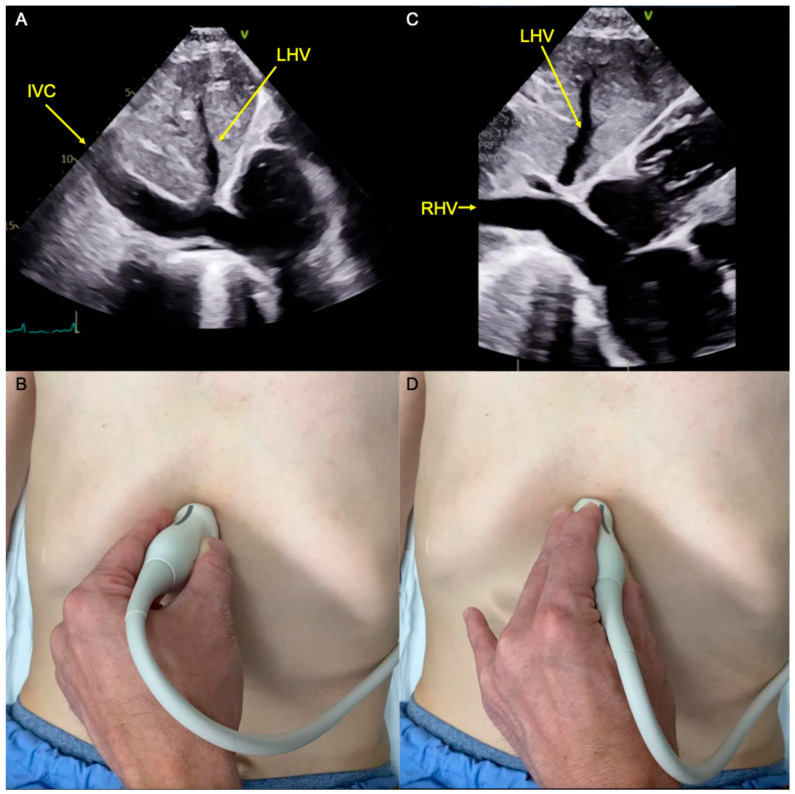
(A) Inferior vena cava (IVC) entering the screen at about 10 o’clock and coursing through the liver before draining into the right atrium. The left hepatic vein (LHV) is seen draining into the IVC at an acute (<90 degree) angle. (B) Demonstration of how Figure 1A was obtained by placing a low-frequency ultrasound probe in the subxiphoid region and aligning the ultrasound beam with the body’s sagittal plane such that the probe indicator pointing is toward the patient’s head. (C) Right hepatic vein (RHV) and left hepatic vein (LHV) making an obtuse (>90 degree) angle as they merge at the hepatic vein confluence. (D) Demonstration of how (C) was obtained by placing the ultrasound probe in an oblique plane such that the probe indicator is pointing approximately toward the patient’s left shoulder.
